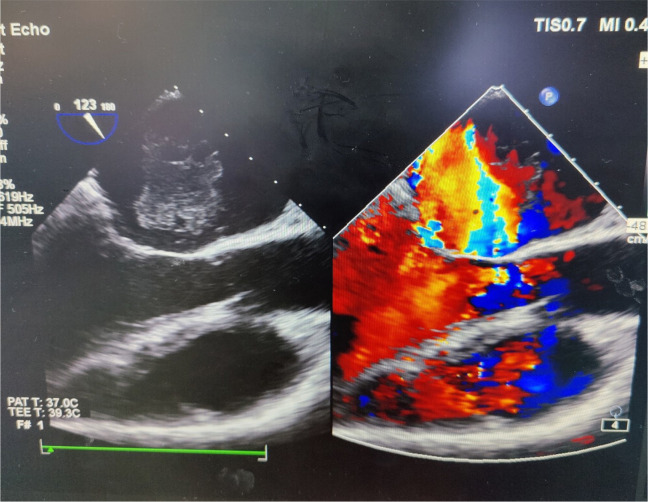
Echocardiogram showing myxoma.
